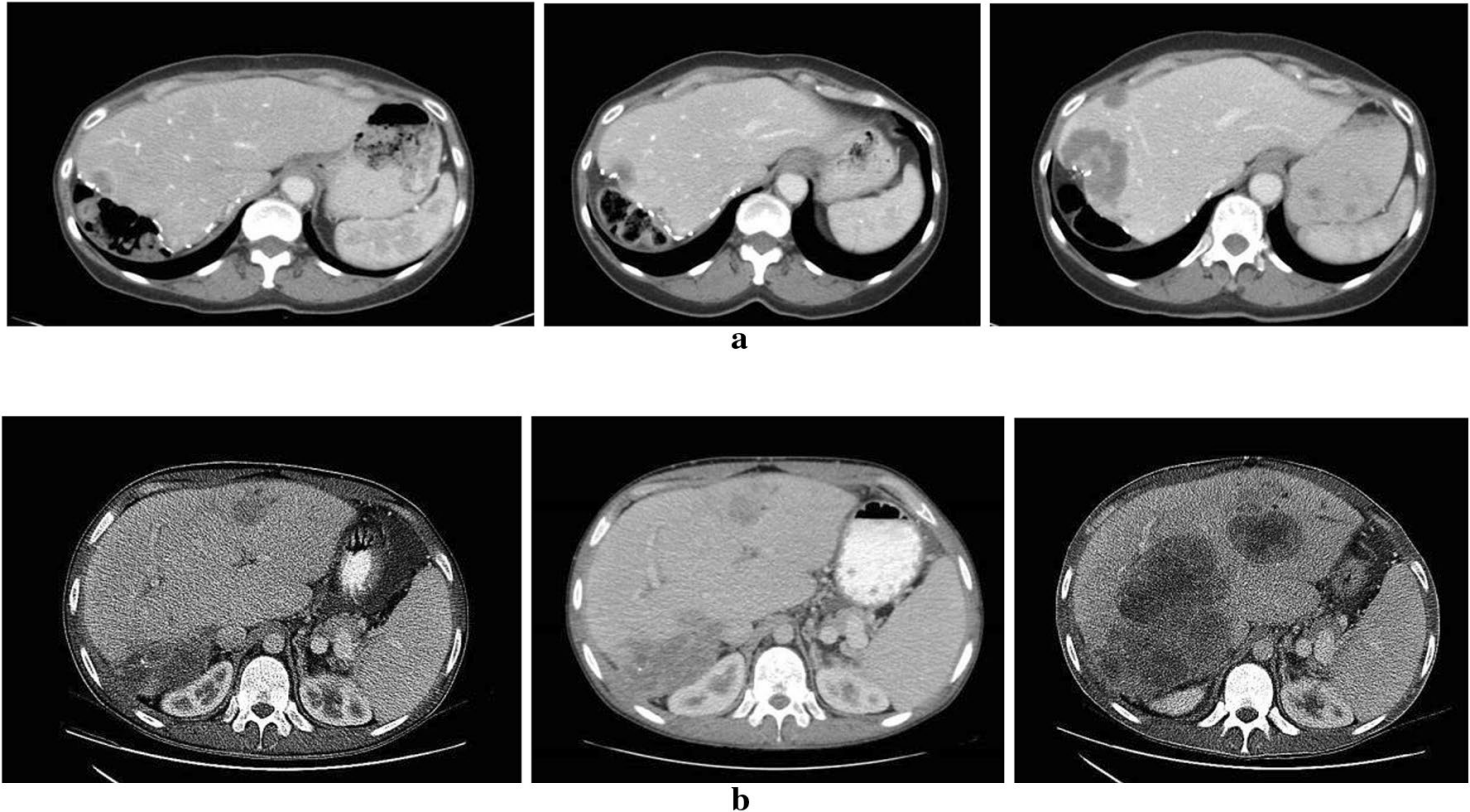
(a) CT-scan of abdomen in patient 102 before baseline (left panel), at baseline (middle panel) and first evaluation at week 8 (right panel). This patient fulfilled the criterion for hyperprogressive disease. (b) CT-scan of abdomen in patient 109 before baseline (left panel), at baseline (middle panel) and first evaluation at wee 8 (right panel). This patient fulfilled the criterion for hyperprogressive disease.
